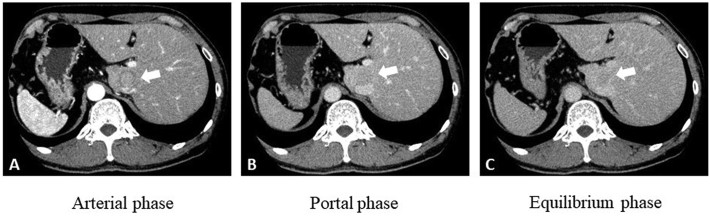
Preoperative computed tomography (CT). A: Arterial phase. B: Portal phase. C: Equilibrium phase. Enhanced CT showed a 2.5 cm nodule at the caudate lobe with early enhancement, followed by washout during portal and equilibrium phase (arrow). The tumor compressed the inferior vena cava dorsally.
